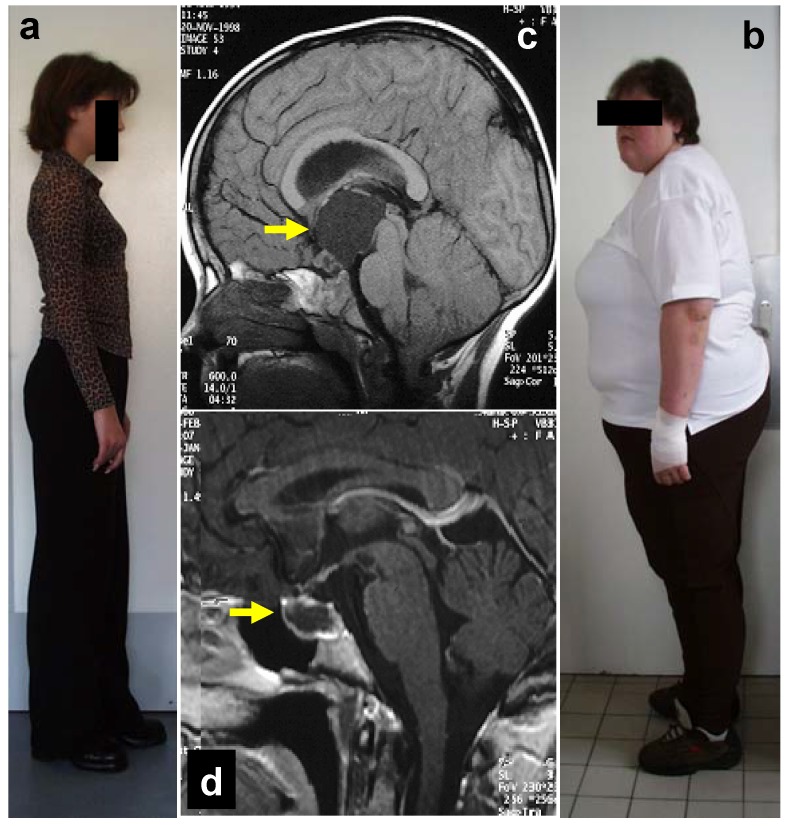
Degree of obesity in relation to the location of childhood-onset craniopharyngioma. In both patients, craniopharyngioma (as indicated by arrow on magnetic resonance imaging before surgery) could be completely resected. Both patients had complete hypopituitarism after surgery requiring endocrine substitution of all hypothalamic-pituitary axes. The patient depicted in (B) developed severe obesity due to hypothalamic lesions of suprasellar parts of craniopharyngioma (C). The patient depicted in (A) presented with a small tumor confined to the sellar region (D). After complete resection, she maintained a normal weight without any eating disorders. Modified from [20], with kind permission of Springer.
